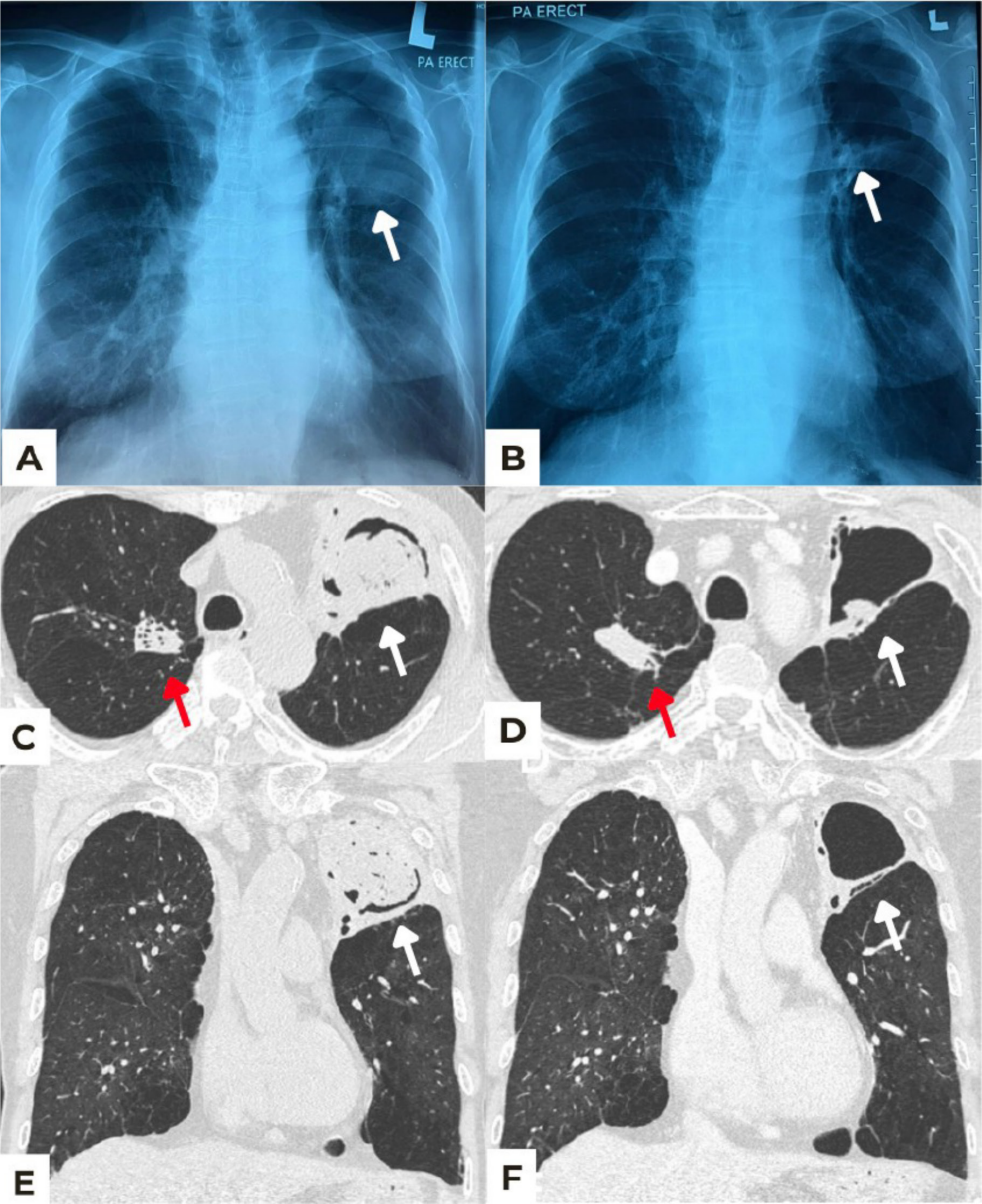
(A) Chest radiograph (PA erect view) demonstrates a well‐defined ovoid opacity with rim of lucent air at its periphery (white arrow), exhibiting an air crescent sign—a classic feature of a fungal ball (aspergilloma) in the left upper zone. (B) Follow‐up chest radiograph (PA erect view) post‐treatment shows marked reduction in the size of the aspergilloma (white arrow), accompanied by increased aeration of the left upper zone cavity, indicating a favourable radiological response to therapy. (C) Axial contrast‐enhanced CT thorax showing thin‐walled cavity in the left upper lobe containing an intracavitary soft‐tissue lesion consistent with a fungal ball (mycetoma) (white arrow) measuring approximately 4.5 × 6.0 × 5.7 cm. There is right apical fibrosis (red arrow) and background emphysematous lung. (D) Follow‐up CT thorax post‐treatment shows significant reduction in the size of the fungal ball within the pre‐existing left upper lobe cavity (white arrow), now measuring approximately 2.6 × 1.2 × 2.2 cm. Persistent right apical fibrosis is noted (red arrow). (E) Coronal reformatted image of C demonstrating the same cavity and fungal ball. (F) Coronal reformatted image of D demonstrating interval reduction in the size of the fungal ball within the left upper lobe cavity.
